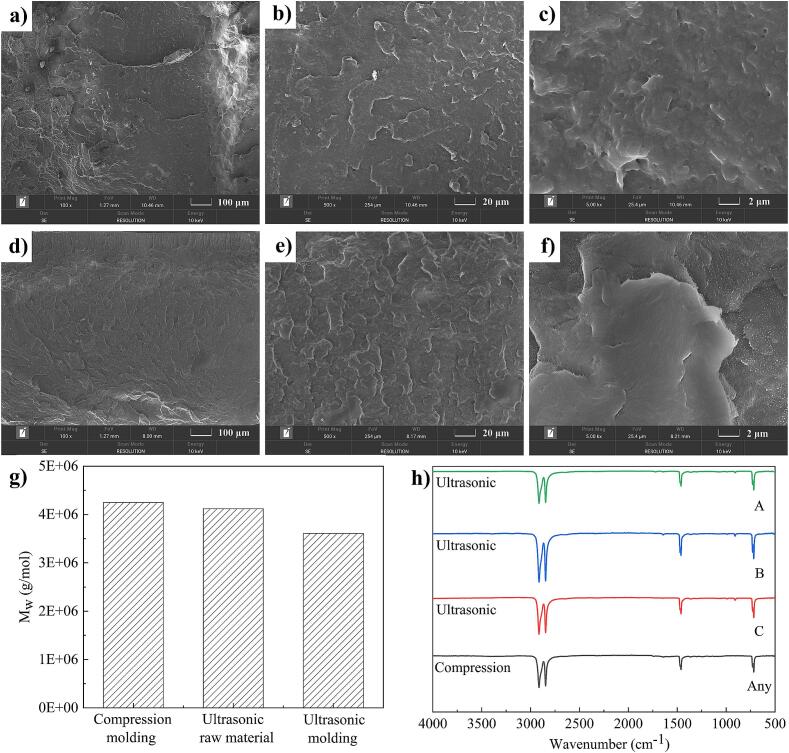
The micro-morphology, molecular weight and FTIR spectra of UHMWPE tensile sample. (a), (b), (c) and (d), (e), (f) The fracture surface micro-morphology of the B region on the tensile sample for compression molding and ultrasonic molding after 100 times, 500 times and 5000 times magnification. (g) The molecular weight measurement results of compression molded tensile sample, ultrasonic plasticizing raw material and C region for ultrasonic molded tensile sample. (h) The FTIR spectra of ABC three regions of ultrasonic molded tensile sample and any region of compression molded tensile sample.
